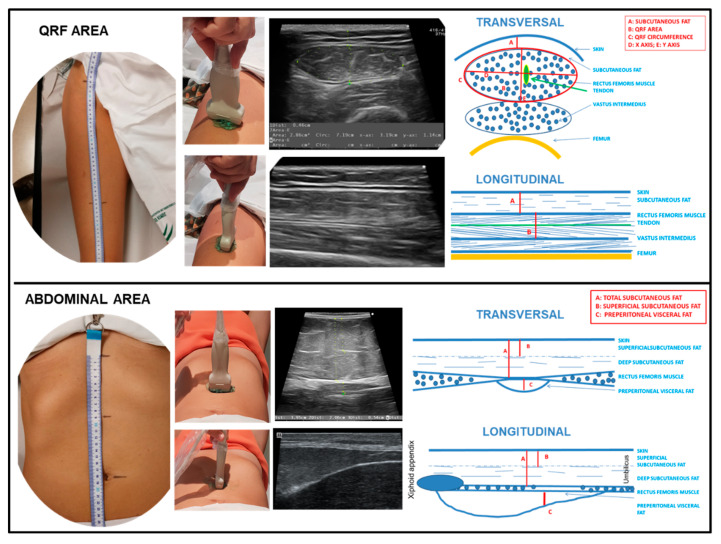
Transversal and longitudinal section of the rectus femoris (QRF) muscle and the abdominal area. Representation of the anatomical measurement area. Position of the ultrasound transducer. Image and scheme of the anatomical structures. To interpret the color references in this figure legend, please see the Web version of this article. Source: own elaboration.
